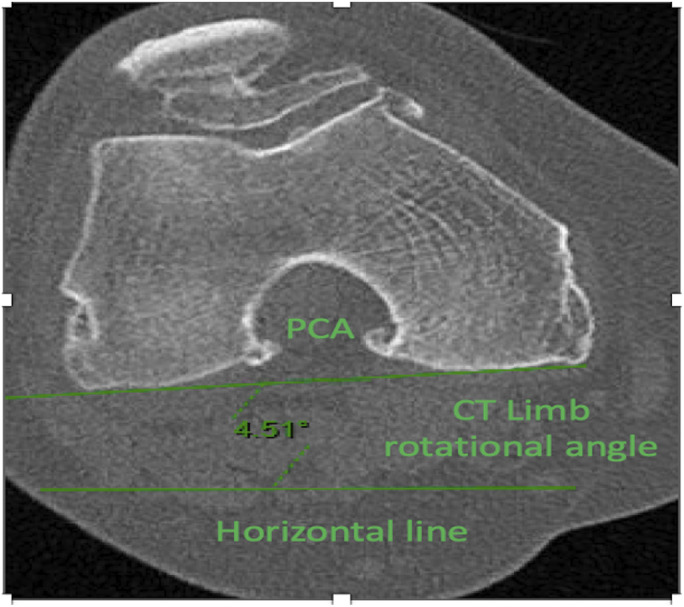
Depiction of the posterior condylar axis (PCA) and CT limb rotational angle (CRA).
